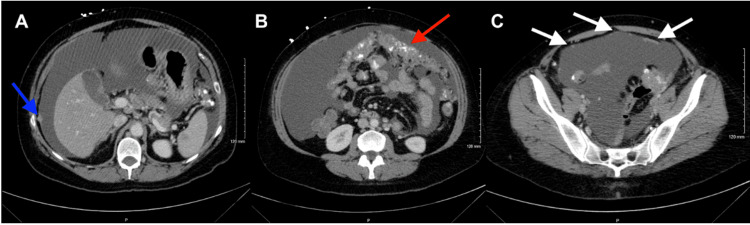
A computed tomography (CT) scan of the abdomen and pelvis was performed on October 5, 2022. A: Evidence of a discrete nodule (blue arrow) in the peritoneum suggests peritoneal carcinomatosis. B: Omental caking (red arrow) with associated calcification of the mesentery suggests adenocarcinoma. C: Anterior peritoneal lining demonstrates nodular enhancement and thickening (white arrows), further indicating carcinomatosis. Ascites is seen throughout the CT images provided, specifically laterally and posteriorly in Figure 1A and B (black fluid within the abdominal cavity).
